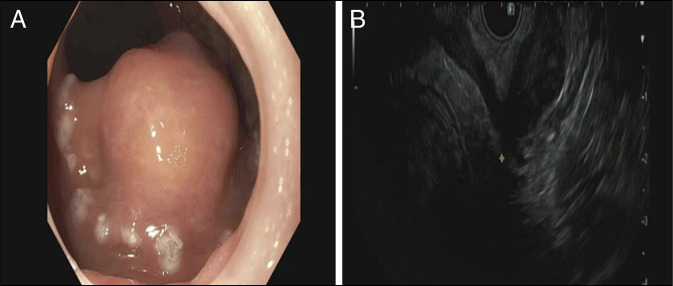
Endoscopic (A) and endoscopic ultrasound (B) view of the 3.1 × 2.1 cm mass along the anterior wall of the distal rectum arising from the muscularis propria.
